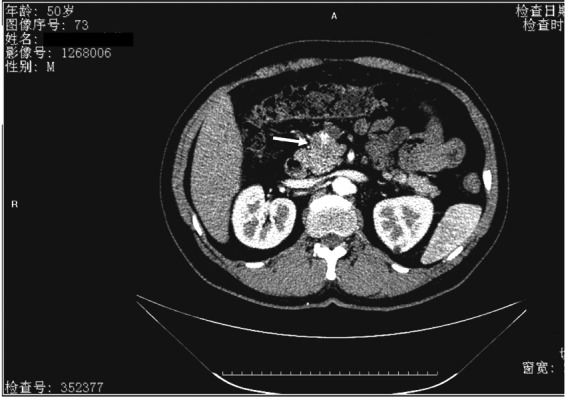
CT scan of a patient with an SPT. Abdominal contrast-enhanced CT scan shows an enlarged pancreatic head containing a well-defined, encapsulated solid cystic mass about 4 cm in diameter (arrow). CT, computed tomography; SPT, solid pseudopapillary tumor.
